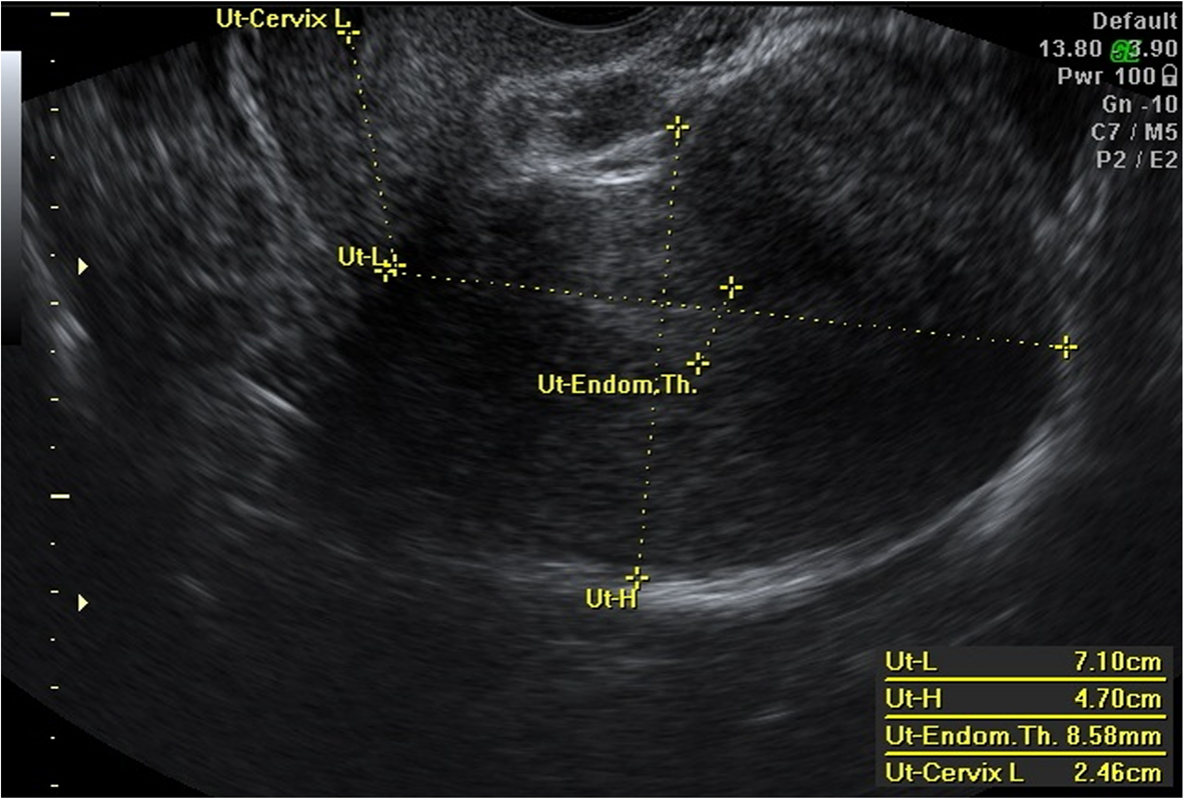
Two-dimensional ultrasound measurement of endometrial thickness
